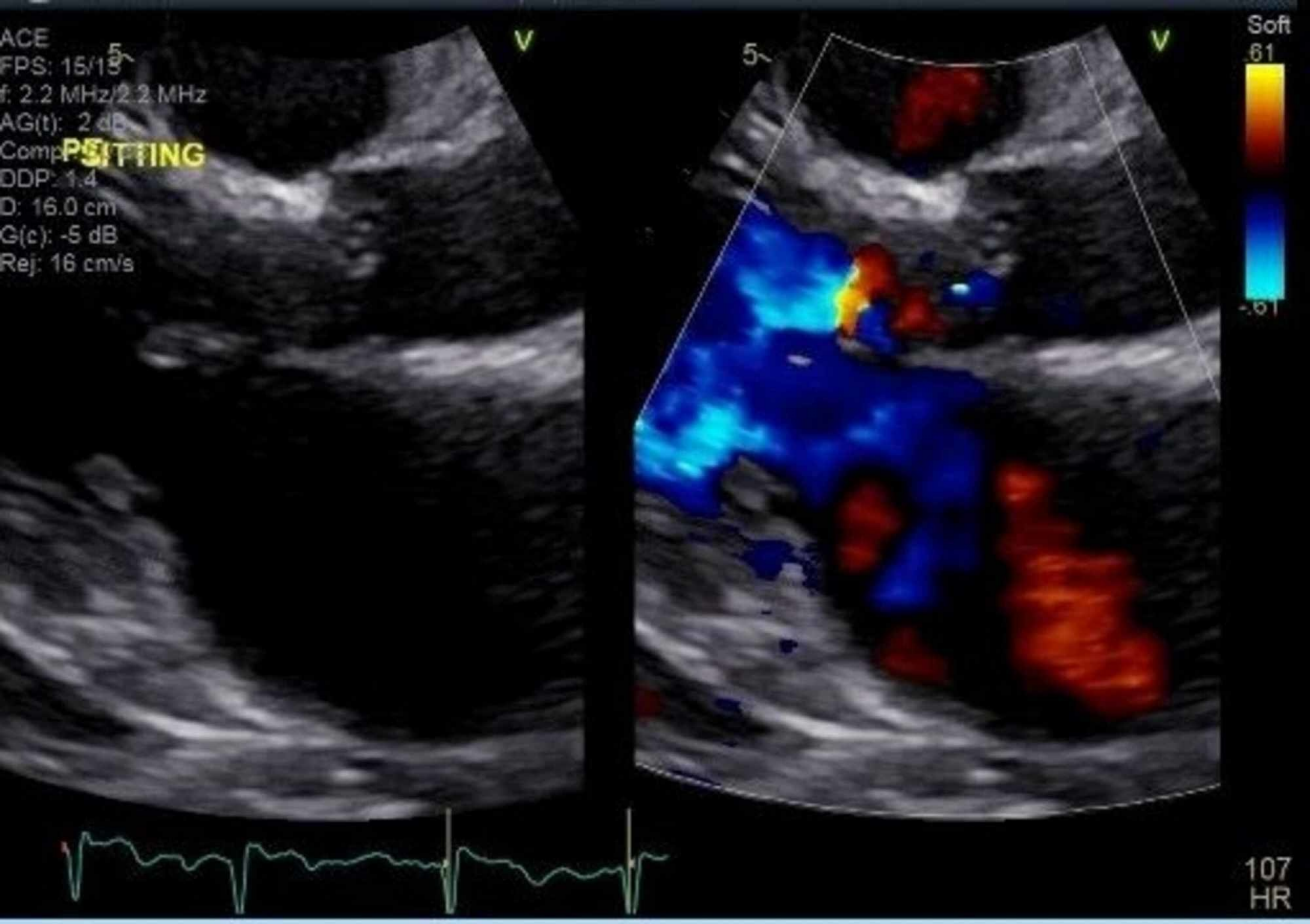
Echocardiography showing normal Left ventricular wall thickness with estimated Left ventricular Ejection Fraction of 55-65% with features consistent with pseudonormal left ventricular filling patter with concomittant abnormal relaxation and increased filling pressure (Grade 2 diastolic dysfunction). Associated aortic and mitral valve regurgitation.
